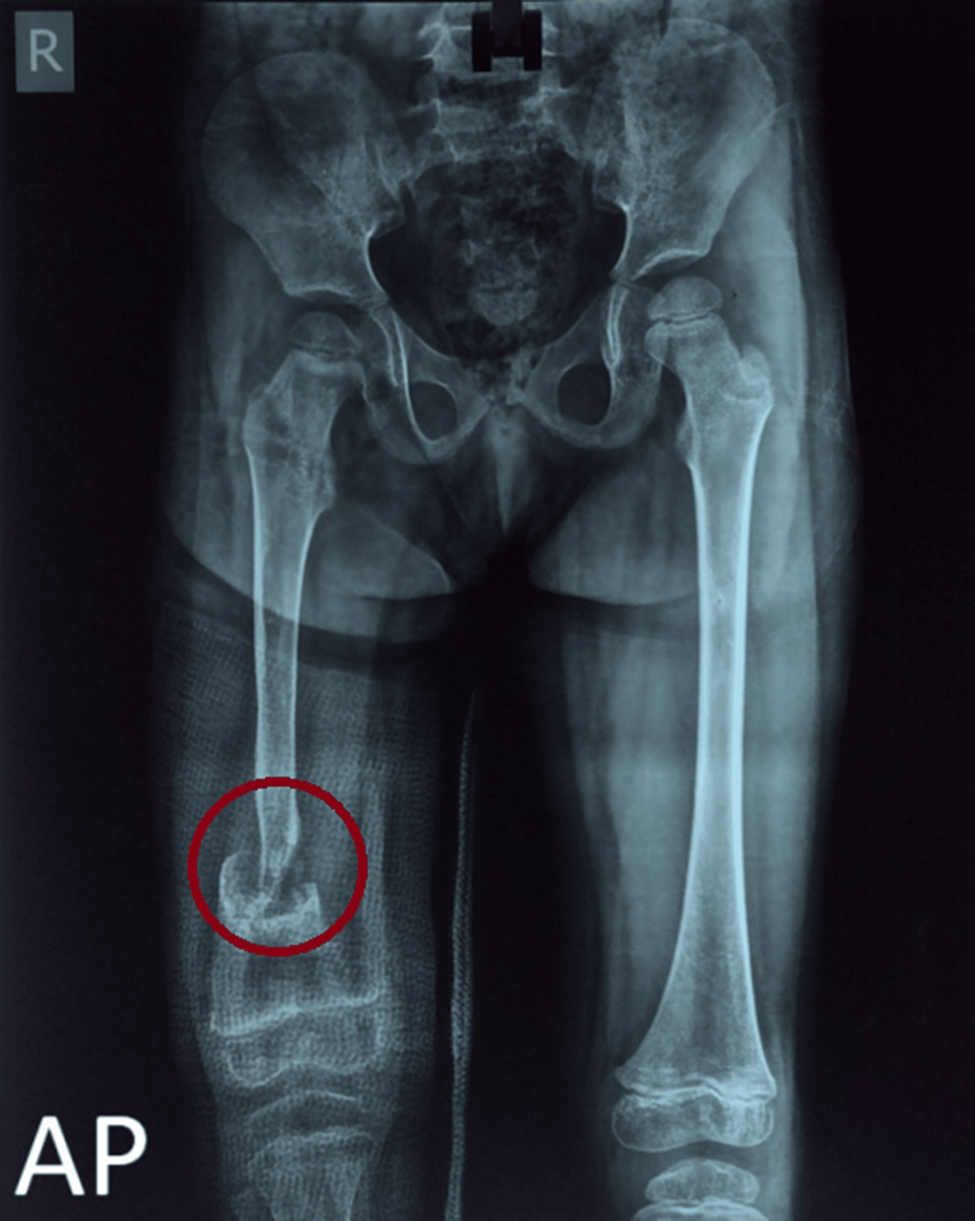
A plain radiograph (AP view) of the pelvis with bilateral hips and a whole-length femur shows pseudoarthrosis of the distal one-third of the right femur
